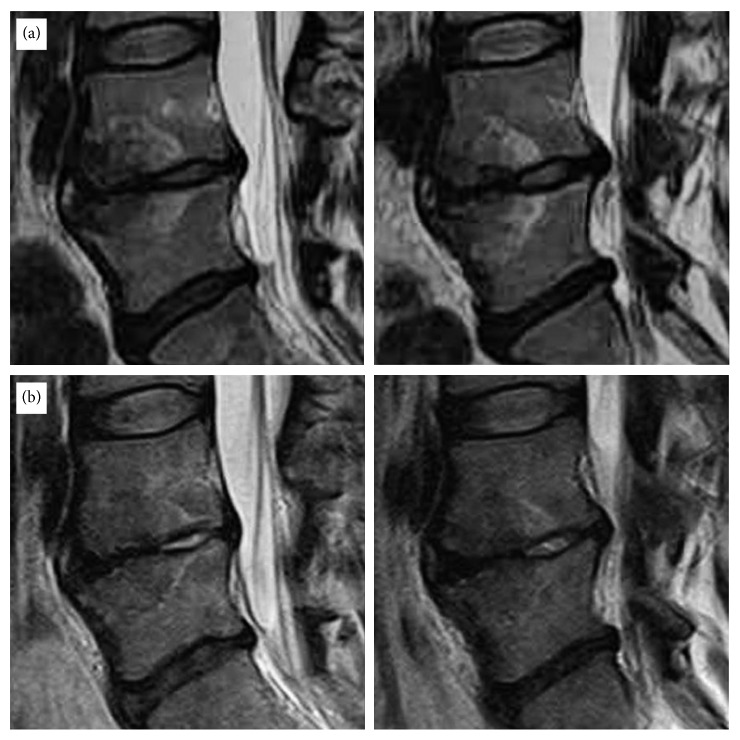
Magnetic resonance imaging (MRI) of a 35-year-old female patient who had suffered severe back pain and bilateral referred leg pain for more than 2 years. This patient's symptom improved significantly after TEBLA at the L4-5 level. The preoperative VAS score of 8 decreased to 1 postoperatively. (a) Preoperative T2WI lumbar magnetic resonance imaging (MRI) sagittal views with Modic Type 1 changes. (b) Postoperative T2WI lumbar MRI sagittal views showing resolution of the Modic reaction.
